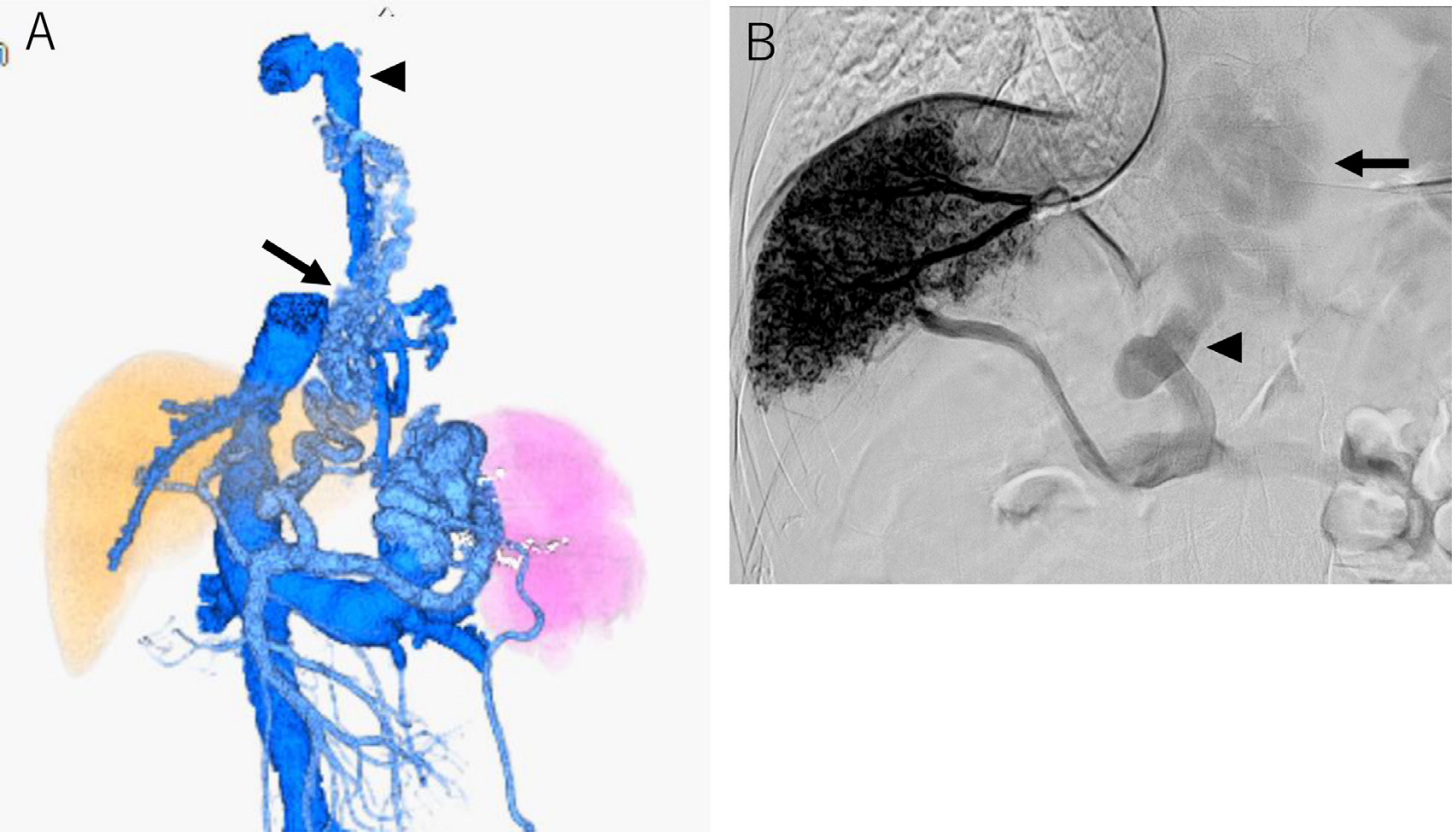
3D-CT reconstruction image and retrograde hepatic venography before EVL. (A) 3D-CT reconstruction image 3 months after the second PSE shows esophageal varices (arrow) and spleen reduced size. The esophageal variceal blood is draining into the azygos vein (arrowhead). (B) Retrograde hepatic venography shows a large left gastric vein (arrowhead) and esophageal varices (arrow).
